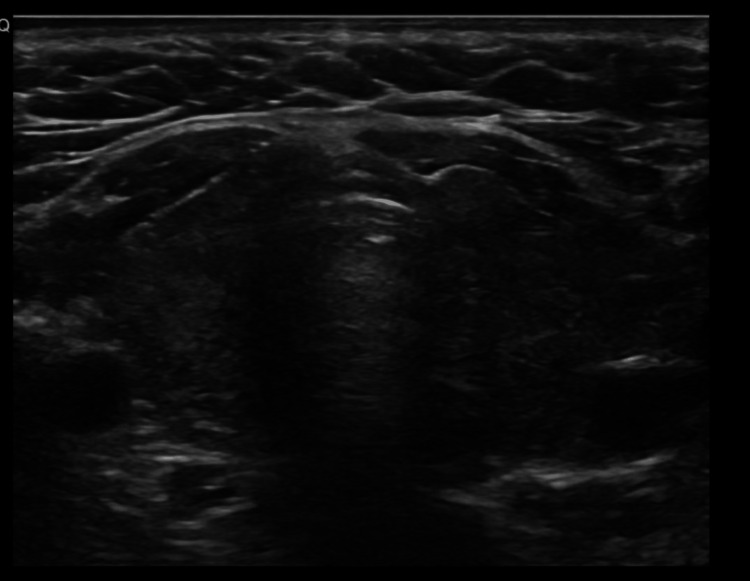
Transverse thyroid ultrasound image showing homogeneous echotexture of both thyroid lobes without nodules
